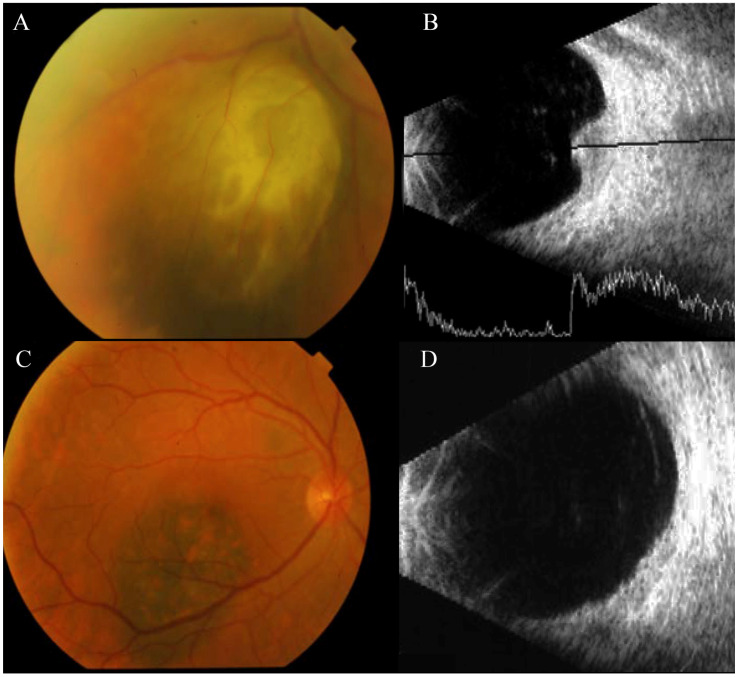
Imaging tests corresponding to the diagnosis of BUM in patient BUM2. (A) Fundus of the eye showing the UM detected in the patient’s left eye. (B) Ocular ultrasound scan carried out on the left eye showing the presence of UM located in the lower nasal quadrant. (C) Fundus of the patient’s right eye, showing a mass located below the fovea compatible with the diagnosis of a small melanoma. (D) Ocular ultrasound scan of the RE of the patient, showing a choroidal mass compatible with a melanoma.
